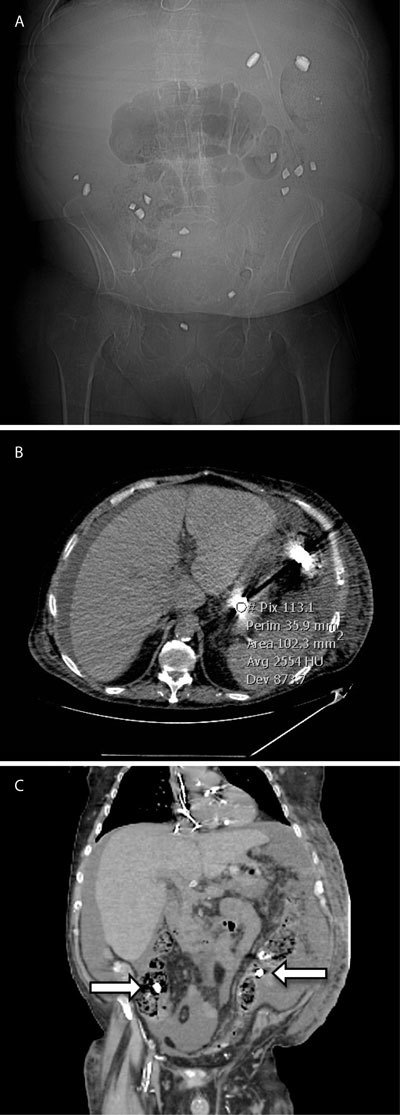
A. Scout examination (prior to the CT acquisition) revealing multiples sharp-edged high-density opacities of random distribution inside the abdomen. B, C.: Computed tomography showing the high density (2500 HU) lanthanum carbonate tablet (arrows) in the stomach and the colon, inducing beam-hardening artifacts.
